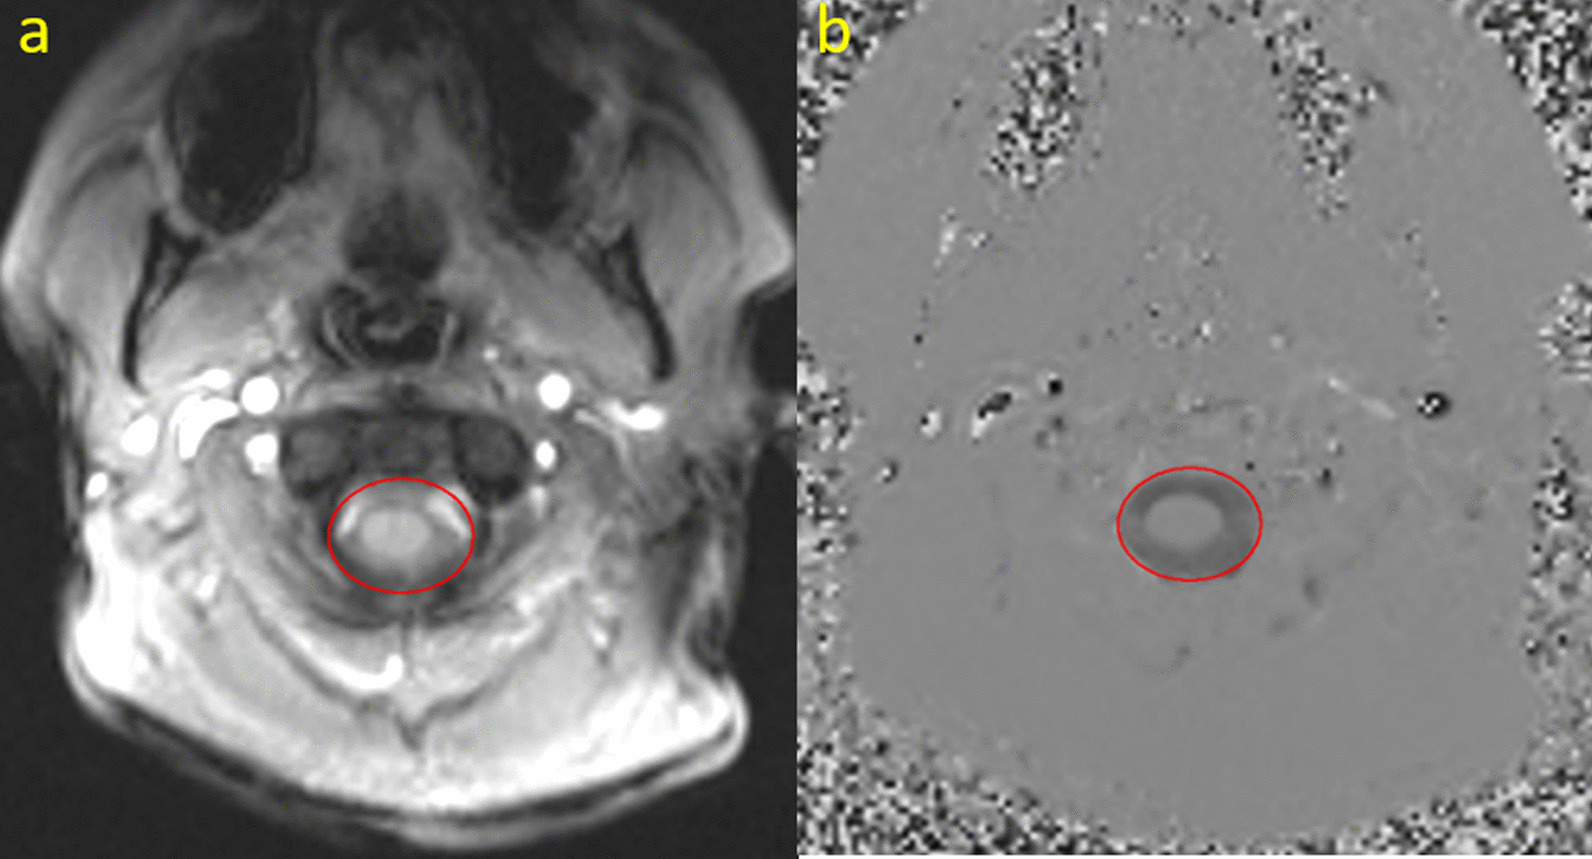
Phase contrast MRI example. *Legend*
